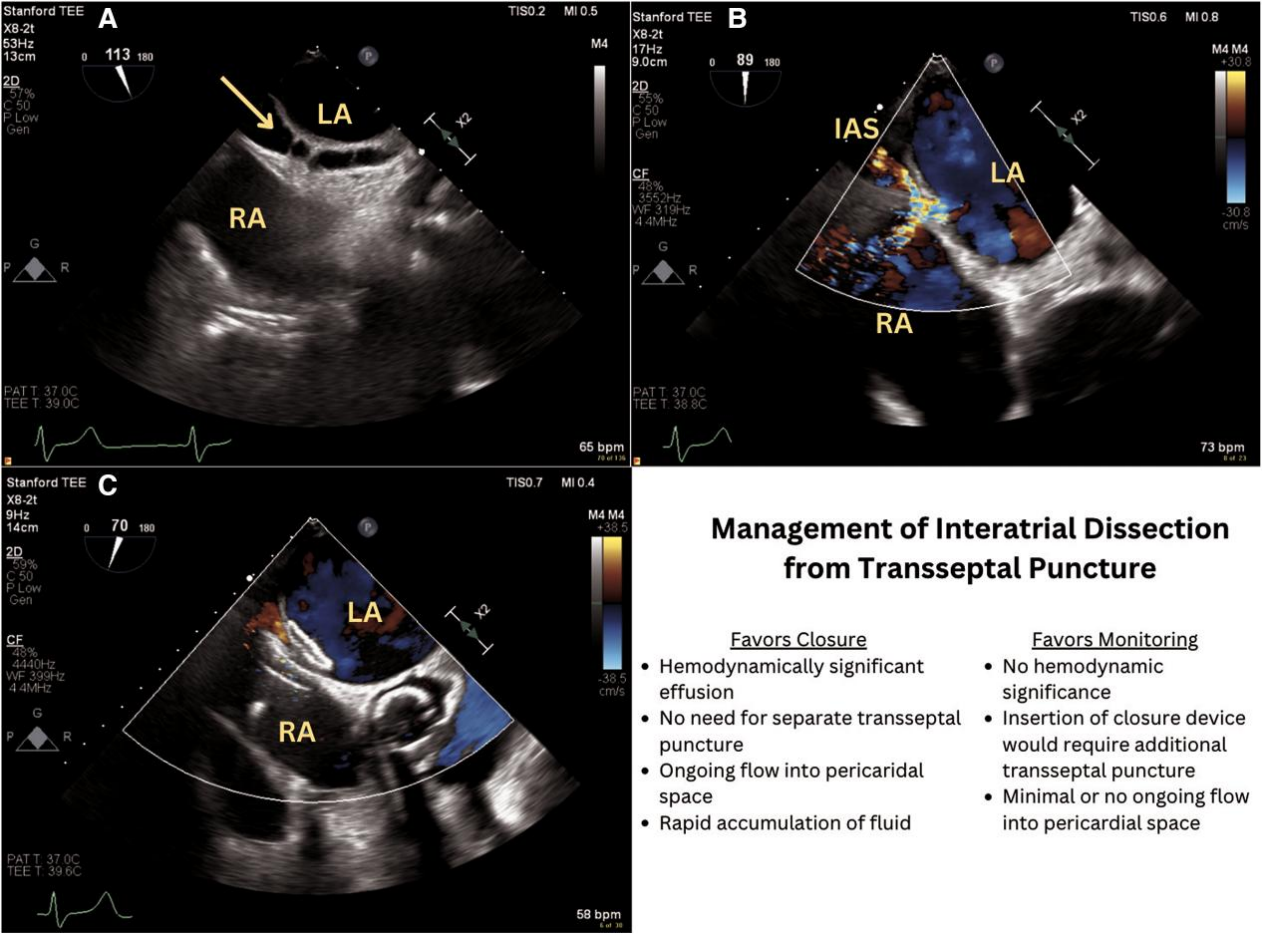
(*A*) Identification of interatrial dissection on transoesophageal bi-caval view (yellow arrow). (*B*) Flow from the left atrium into both the right atrium and interatrial dissection on modified bi-caval view. (*C*) Reduction of flow from LA into RA and IAS following placement of Amplatzer closure device across defect on modified bi-caval view. IAS, interatrial septum; LA, left atrium; RA, right atrium.
